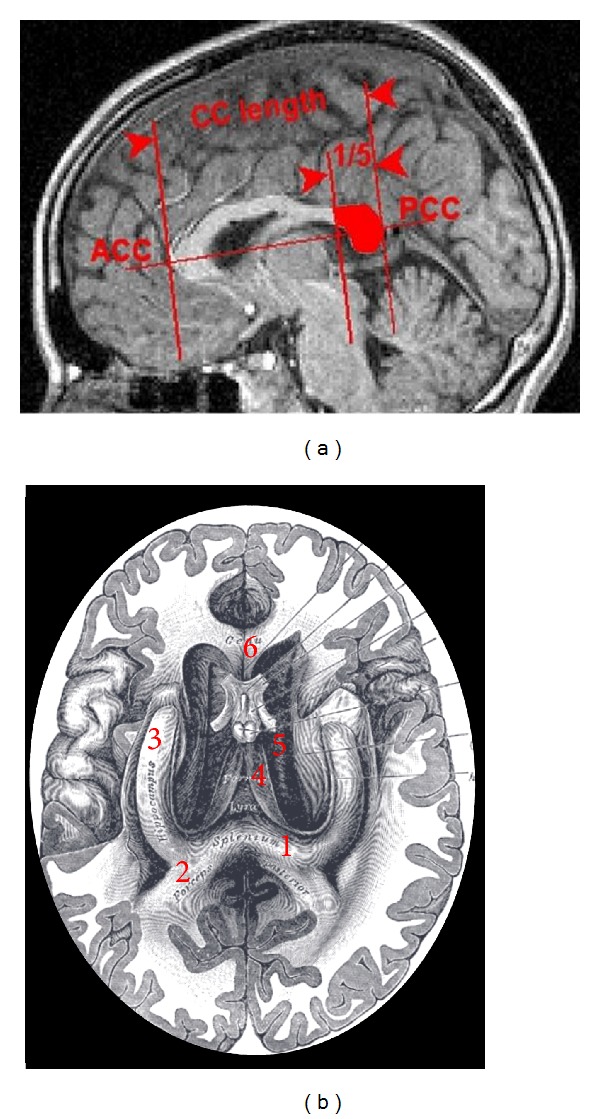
Midsagittal and axial views of the splenium. (a) Midsagittal T1-weighted MRI shows the corpus callosum (CC) and the splenium (in red). According to the conventional partitioning scheme, the splenium corresponds to the posterior 1/5 of the CC, which is separated by the border line perpendicular to the line linking the most anterior (ACC) and posterior (PCC) points of the CC. (b) Axial view of the splenium (1) from Gray's Anatomy of the Human Body. The numbers refer to the posterior forceps (2), hippocampus (3), fornix (4), undersurface of the CC (5), and genu of internal capsule (6).
